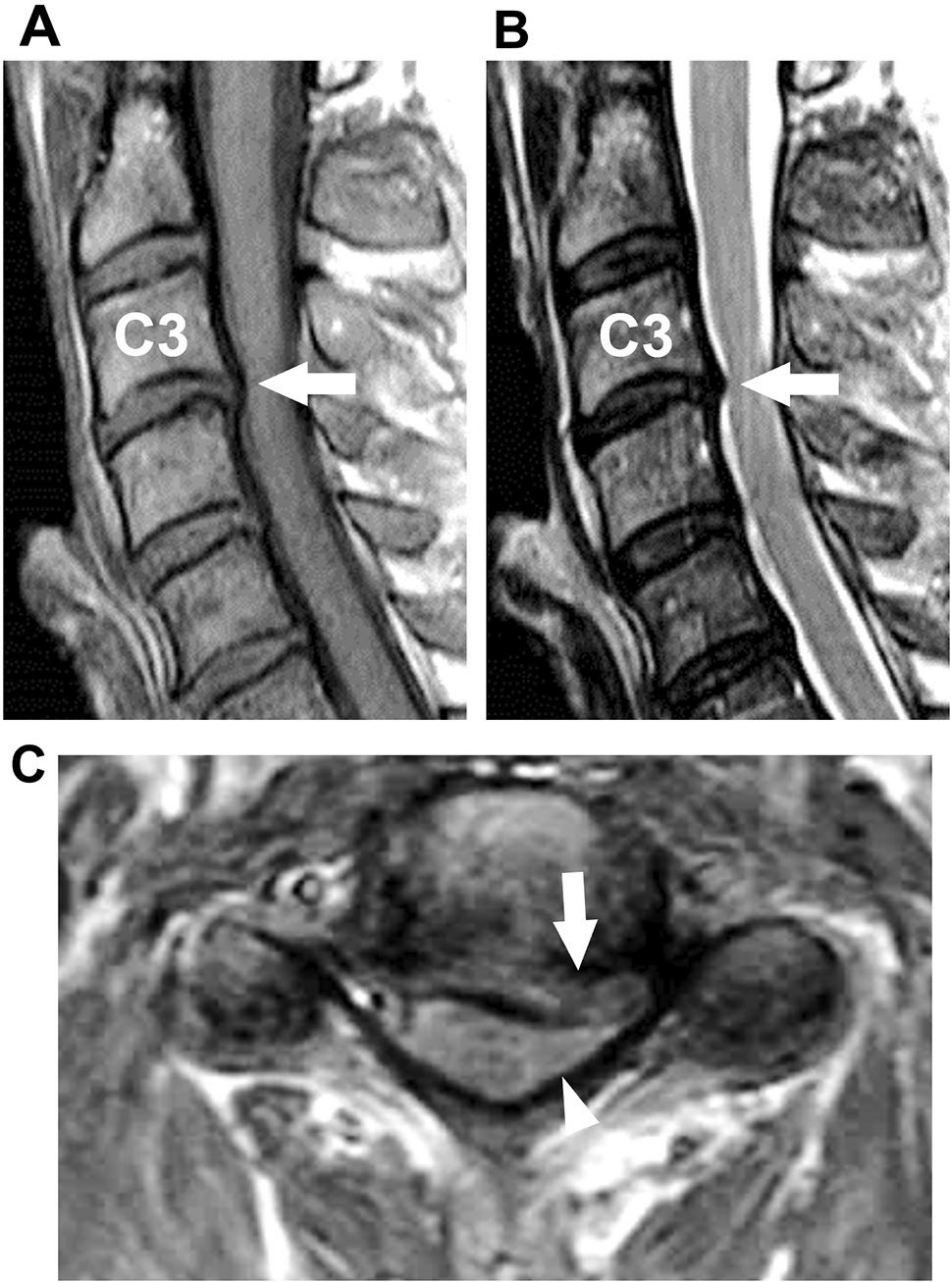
Cervical cord compression on MRI. (A) T1- and (B) T2-weighted sagittal images of 50-year-old man with CSM show posterolateral disk protrusion at C3/4 levels producing concave defect in cervical cord (arrow). (C) T1-weighed axial image at C3/4 level shows posterolateral disk protrusion (arrow) compressing spinal cord (arrowhead). *CSM* cervical spondylotic myelopathy.
